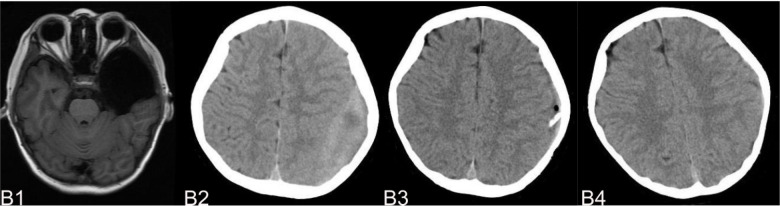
(B) shows a typical patient, who had a subdural hemorrhage during the follow-up time after operation. B1 shows the IAC MRI image before operation. B2 demonstrates the IAC hemorrhage after head trauma 2 months after operation. B3 shows the surgical treatment towards the hematoma. B4 shows the recovery of the subdural hemorrhage after the surgical treatment.
